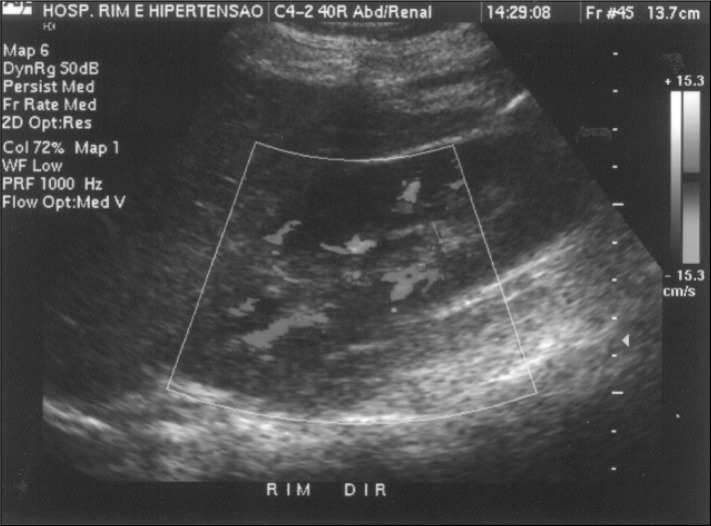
Right renal Doppler ultrasound showing inferior cava thrombosis including the area of the junction of the renal veins.
